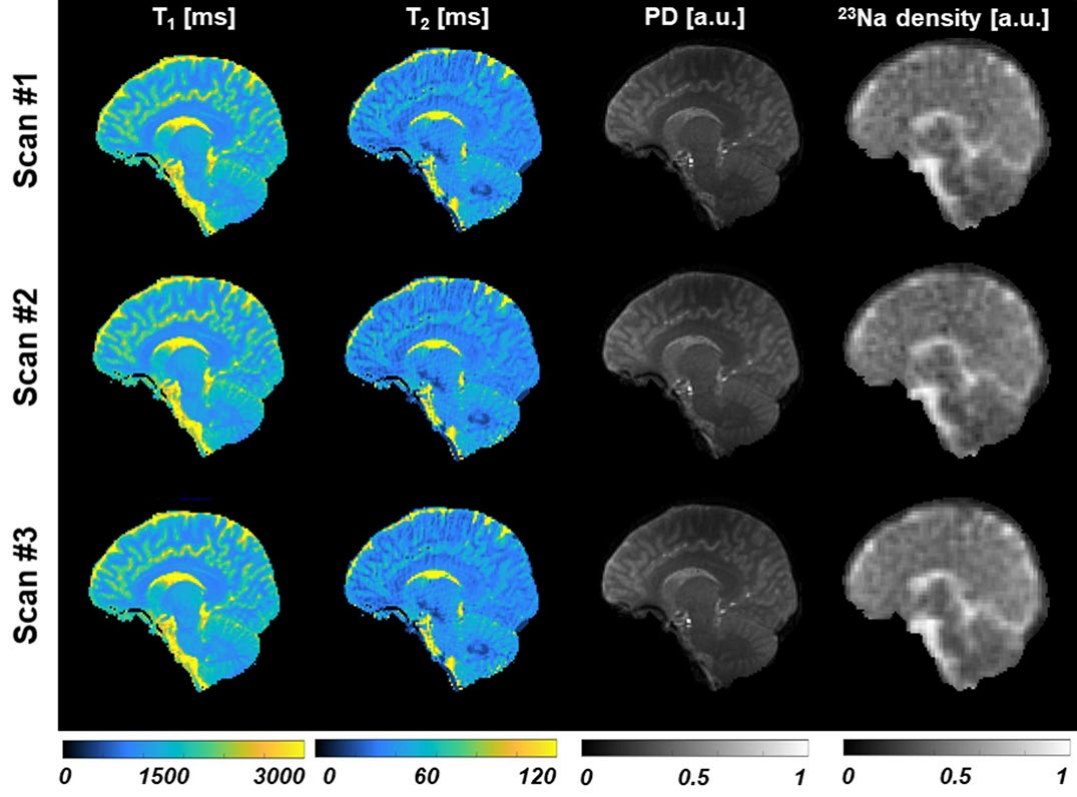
Maps from the 3 scans acquired on subject 1 (after co-registration and masking) with simultaneous ^1^H MRF/^23^Na MRI. The in-plane resolution is 1.5 × 1.5 mm^2^ for the proton images and 2.85 × 2.85 mm^2^ for the sodium image. Slice thickness is 3 mm for both nuclei. PD is proton density.
